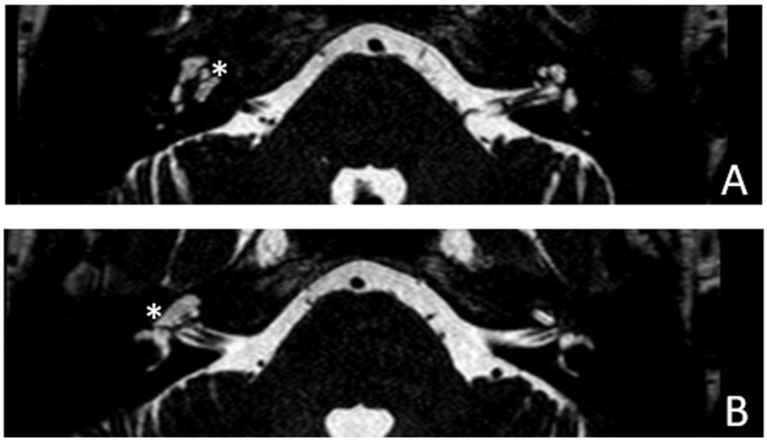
MR images of patient #5 with otosclerosis. MR cisternography. (A,B), axial plane. Note the presence of fluid signal in the newly formed cavity on the right side only (white asterisks).
